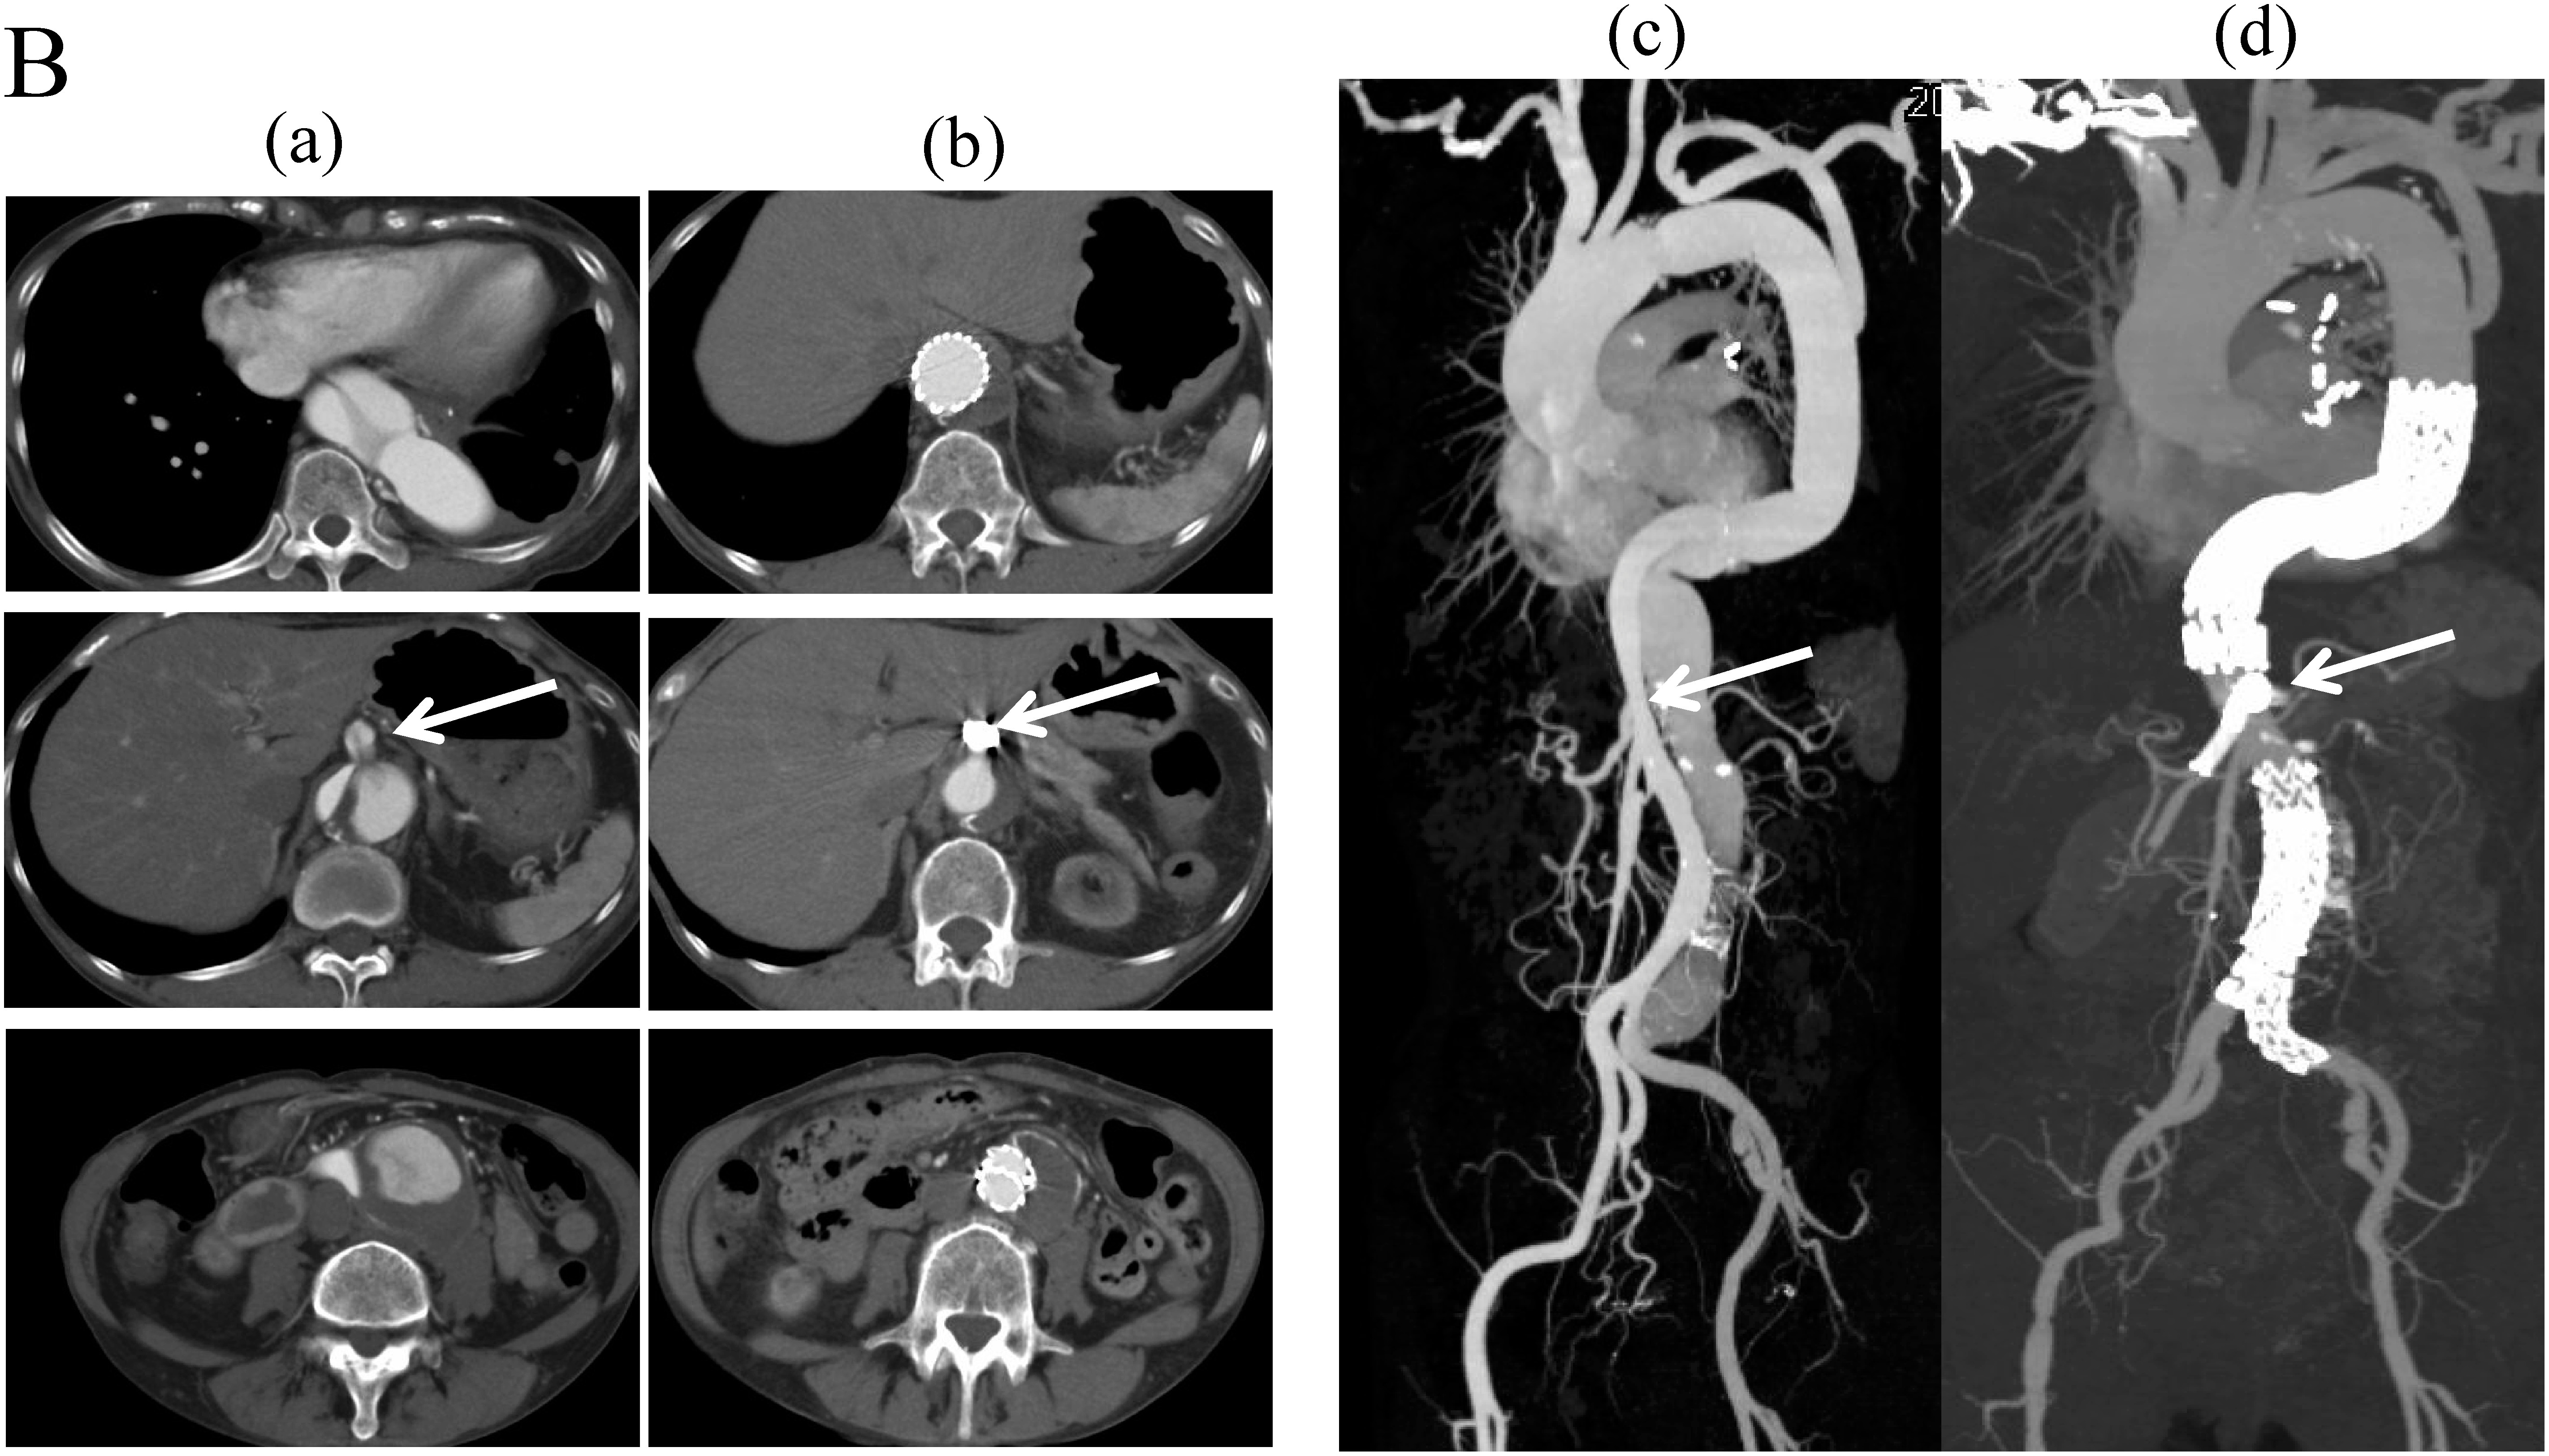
Fig. 1B A woman in her 50s after being treated with descending aorta replacement after chronic type B aortic dissection. (a), (c) Preoperative contrast enhanced computed tomography (CECT; axial and 3-dimensional) shows reentry points in the distal anastomosis, celiac trunk, lumber artery, left common iliac artery. (b), (d) CECT three years after thoracic endovascular aortic repair with reentry closure shows complete false lumen thrombosis and the reduction of false lumen area.
